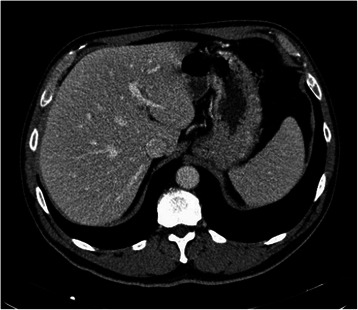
Contrast enhanced abdominal preoperative staging CT showing no liver nodules.
